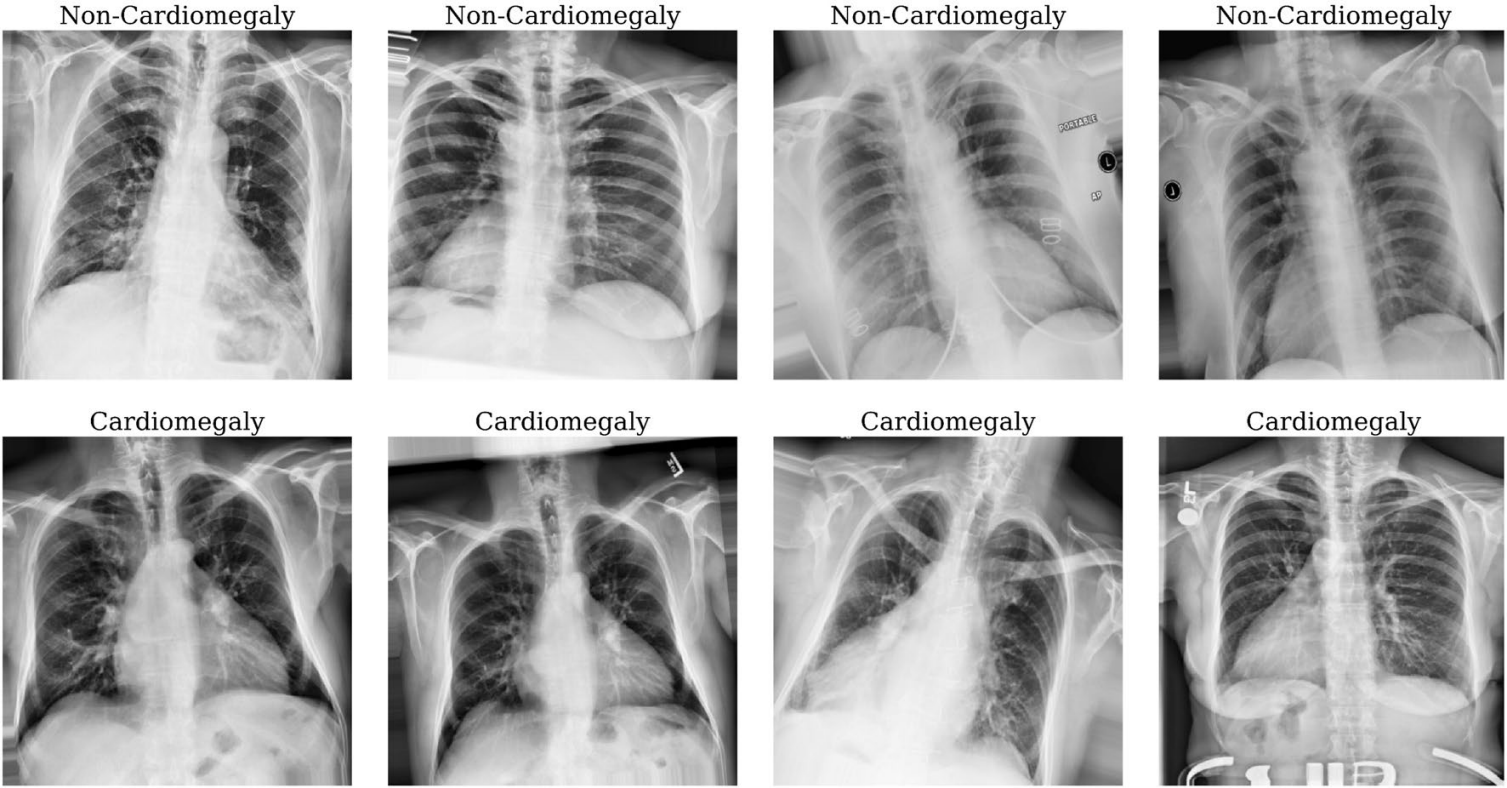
Pre-processed and augmented x-ray images used as training samples. The methods used for augmentation are shifts, rotations, flips, shear, brightness, and zooming. Cardiomegaly and the healthy images were mapped as 1 (Cardiomegaly) and 0 (non-Cardiomegaly) respectively.
